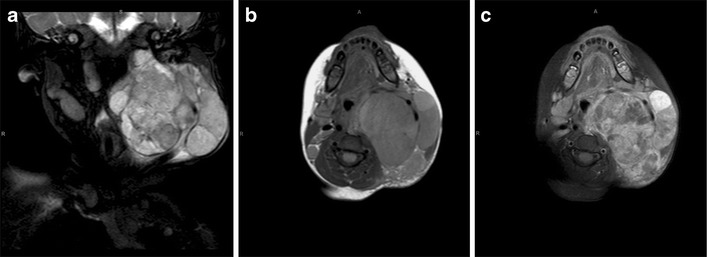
Yolk sac tumour with rhabdoid elements. Coronal T2-W (a) and axial T1-W (b) MRI show a large well-circumscribed left cervical mass centered in the parapharyngeal space, extending from the skull base to the level of the thoracic inlet, with associated right cervical adenopathy. The mass is predominantly hyperintense on T2-W imaging (a) and demonstrates heterogenous post-contrast enhancement (c)
